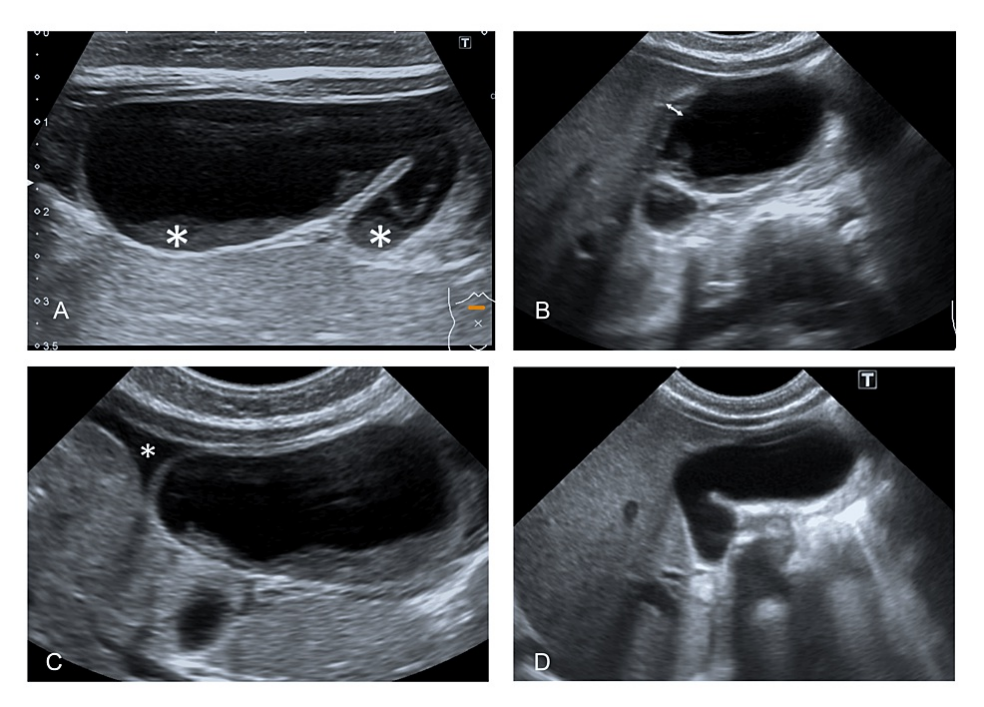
Hepatobiliary ultrasound A) Marked dilatation of the gallbladder with internal bile sludge; B) Gallbladder wall thickening; C) Pericholecystic fluid; D) Resolution of the previously detected distended gallbladder and internal bile sludge without pericholecystic fluid.
